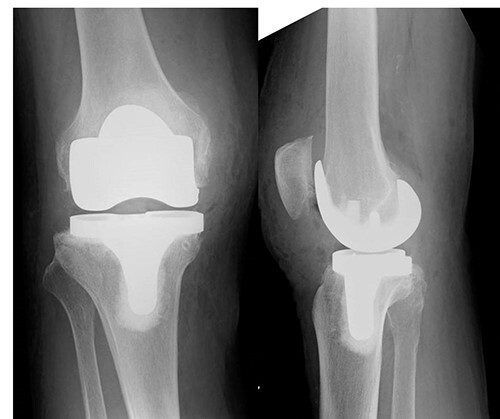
X-ray, 3 months post-operatively, did not show any patellar alta.
